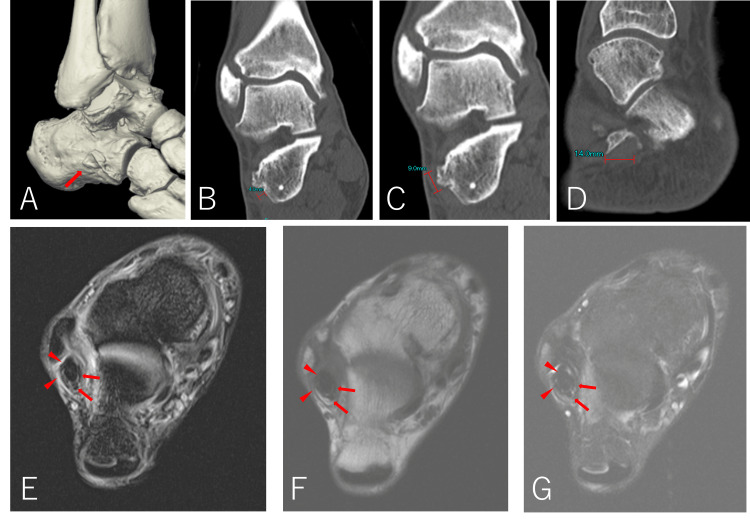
Preoperative images (A-D) Three-dimensional and plane computed tomography scan showing a 9 × 14 × 4 mm osteophyte at the peroneal tubercle(arrow). (E) T1-weighted, (F) T2-weighted, and (G) Short tau inversion recovery axial magnetic resonance image showing a thinned peroneus brevis tendon (arrow) and a hypertrophied peroneus longus tendon (arrowhead).
